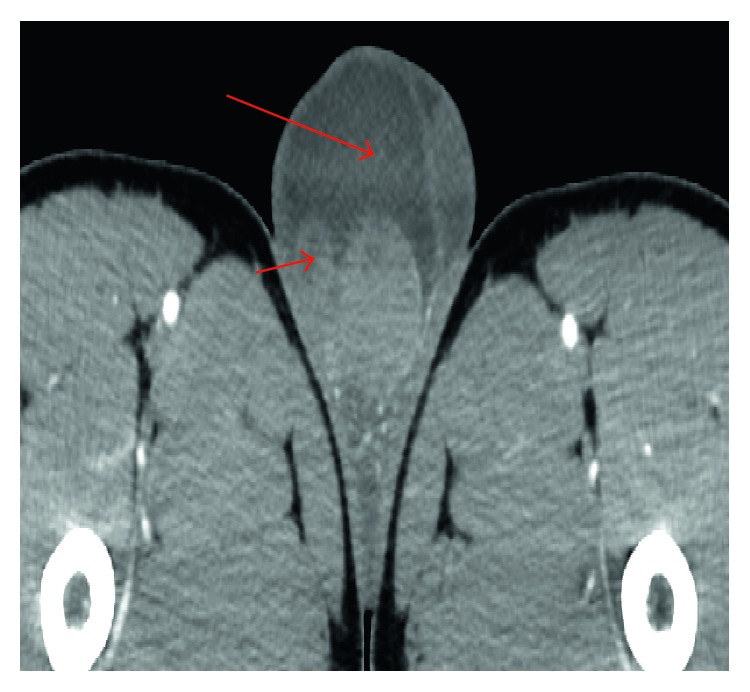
Axial computed tomography (CT) image, depicting a heterogeneous right testicle, with associated right scrotal fluid collection (long arrow) and inflamed right epididymis (short arrow).
